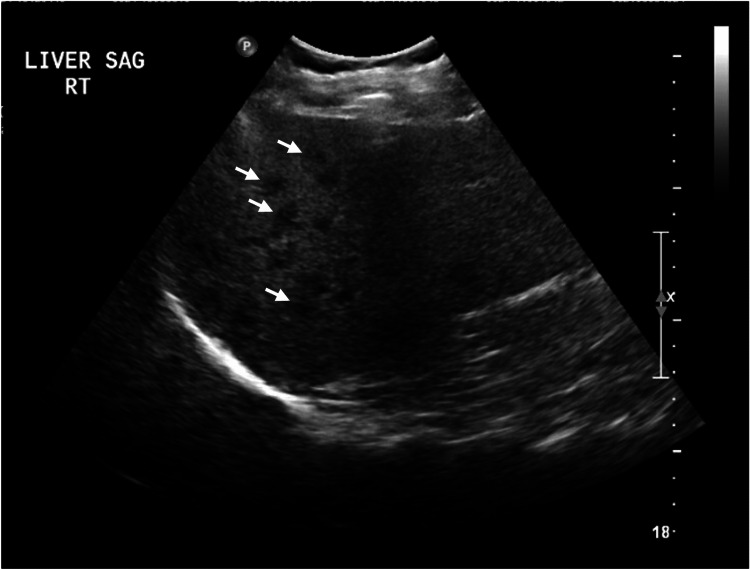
Liver Findings on Ultrasound Imaging Ultrasound demonstrated liver enlargement (17.7 cm) with areas of diffuse hypoechogenicity throughout the liver parenchyma suggestive of fatty infiltration (arrows).
